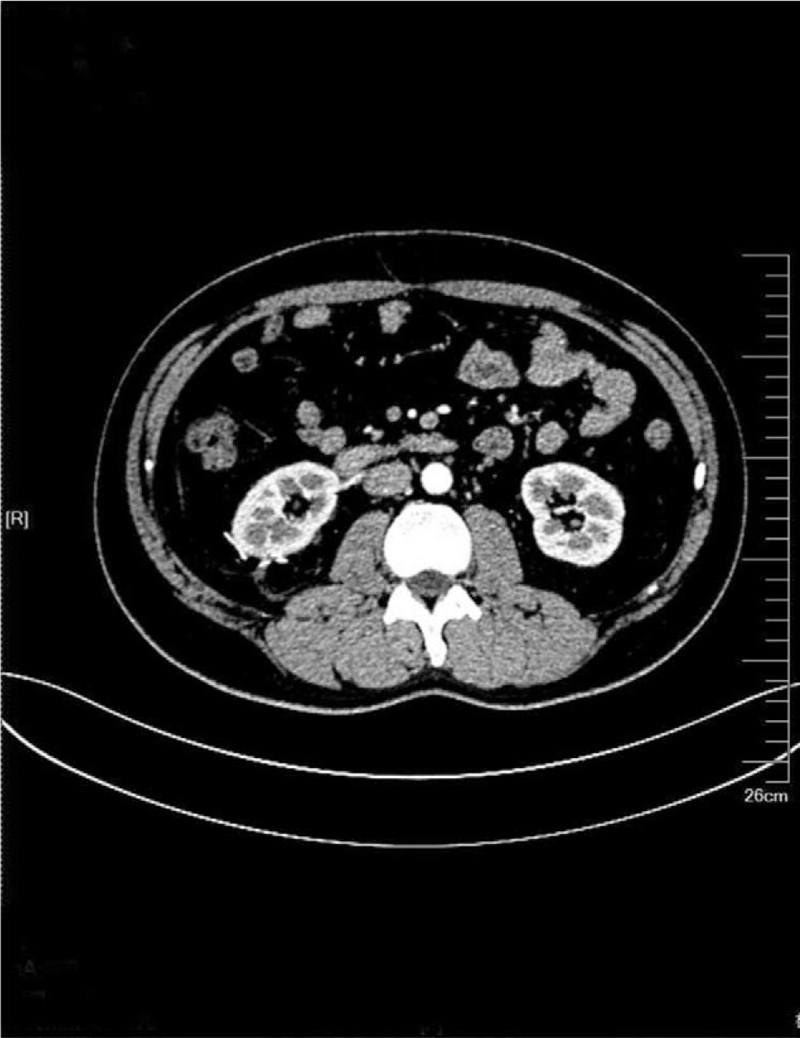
CT abdomen: no obvious signs of recurrence.
